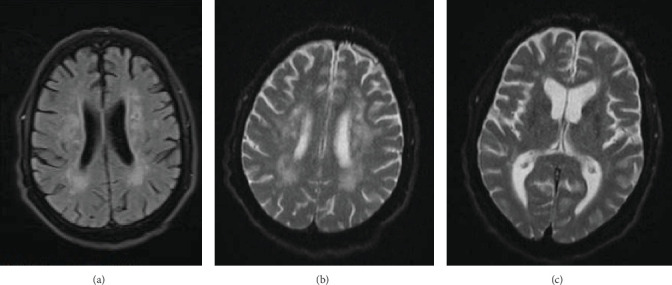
Magnetic resonance imaging (MRI): bilateral calcification of basal ganglia, pulvinar region of thalami, and dentate nuclei (A, B). Bilateral old lacunar infarct in centrum semiovale and bilateral abnormal signal intensity foci in the periventricular white matter and centrum semiovale (C).
